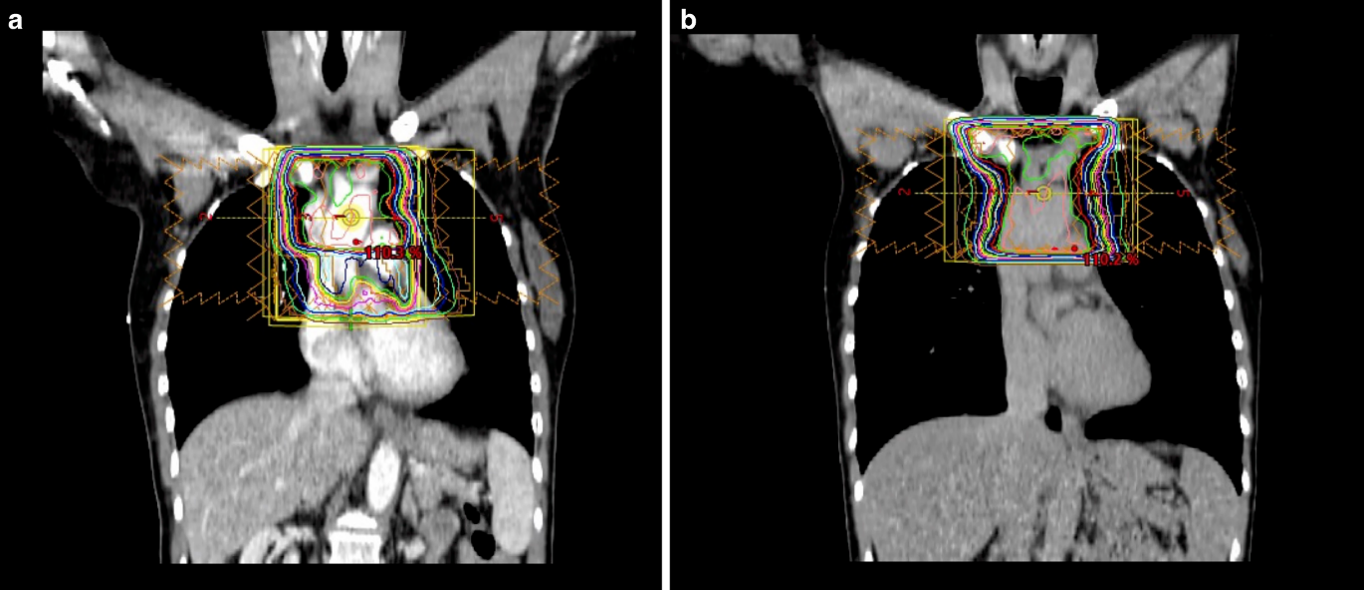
Deep inspiration breath-hold technique for mediastinal lymphoma. Frontal CT scans of the same patient with a mediastinal lymphoma residue prior to radiotherapy (RT) with isodose lines of a planned intensity-modulated RT (IMRT) plan. Scans were acquired in a free breathing and b deep inspiration breath-hold. Note the inflation of the lungs and the displacement of the heart from the target volume, enabling better sparing of these organs at risk
